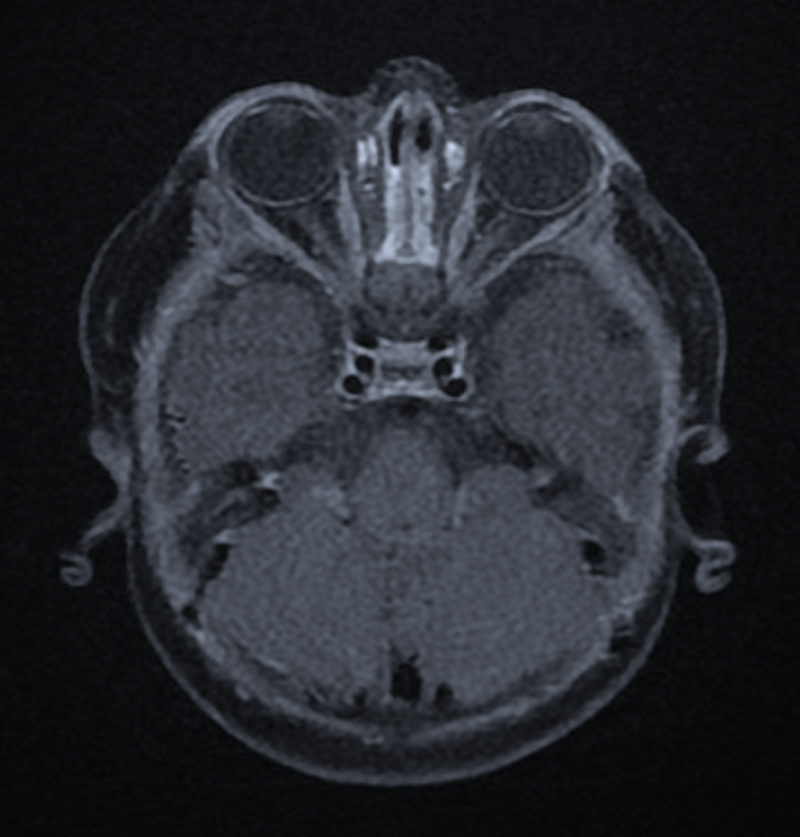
Magnetic resonance imaging axial T1-weighted: narrowed optic canals.
